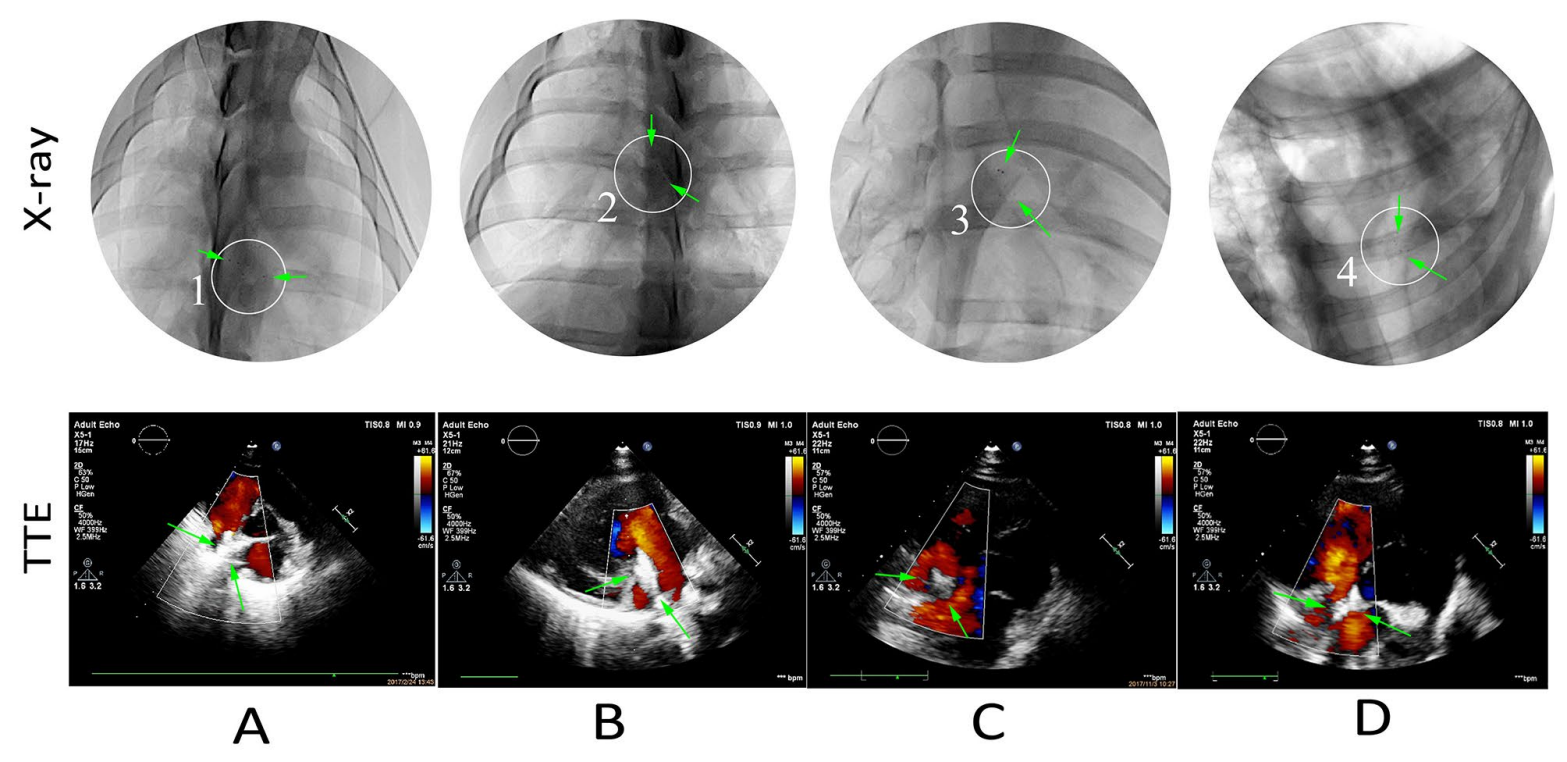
Postoperative examinations of PLLA occluders by X-ray and transthoracic echocardiography (TTE). (A) 3 months after implantation. (B) 6 months after implantation. (C) 2 months after implantation. (D) 24 months after implantation. Green arrows indicated the PLLA ASD occluder. PLLA: poly L-lactide.ASD: atrial septal defect
